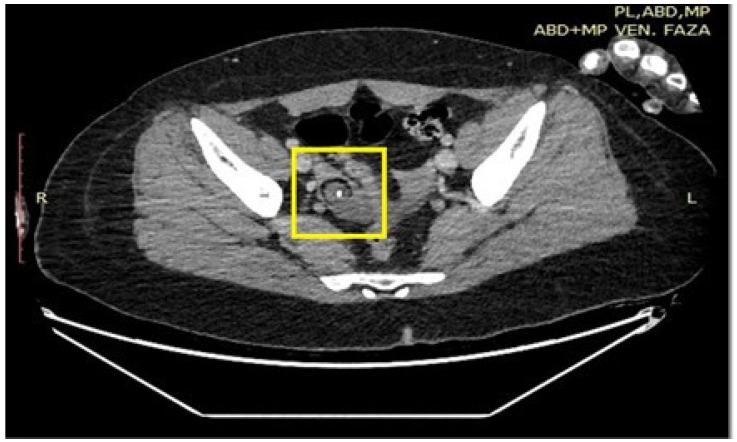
CT examination of the small pelvis, with a dermoid cyst (yellow box) found in the region of the right ovary.
